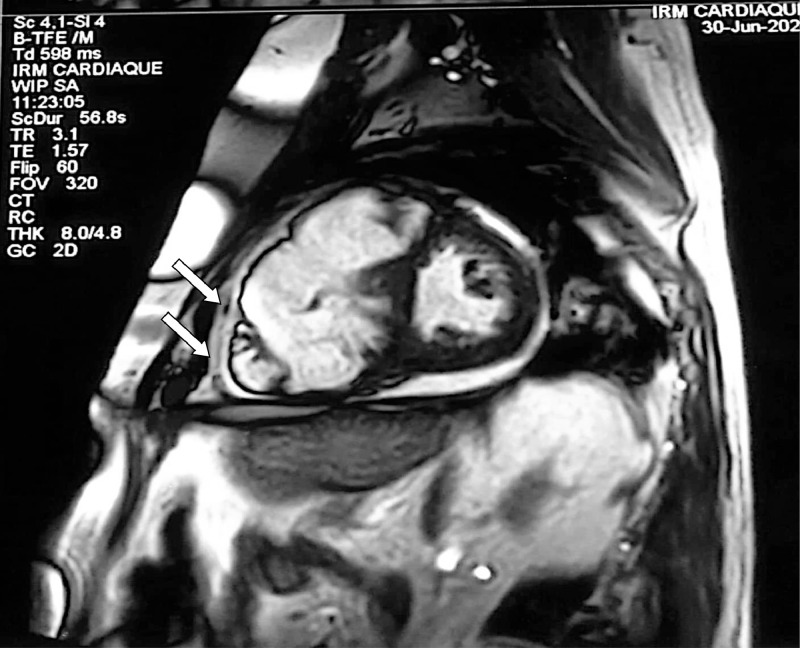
Cardiac MRI sequence Cine-MRI short-axis sequence showing RV dilatation with trabeculations of the apex of the RV (white arrow).
